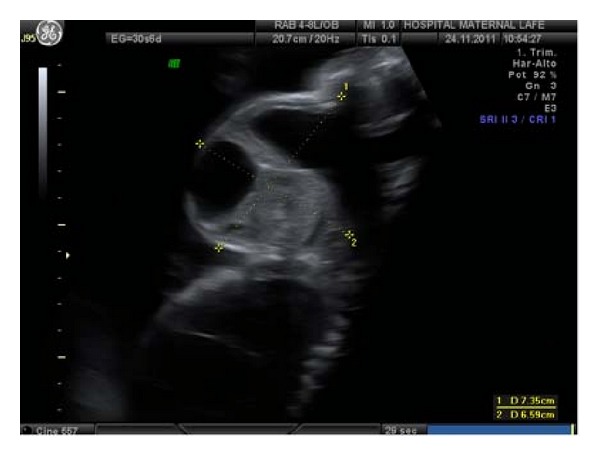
Lateral view of the last ultrasound control before surgery.
